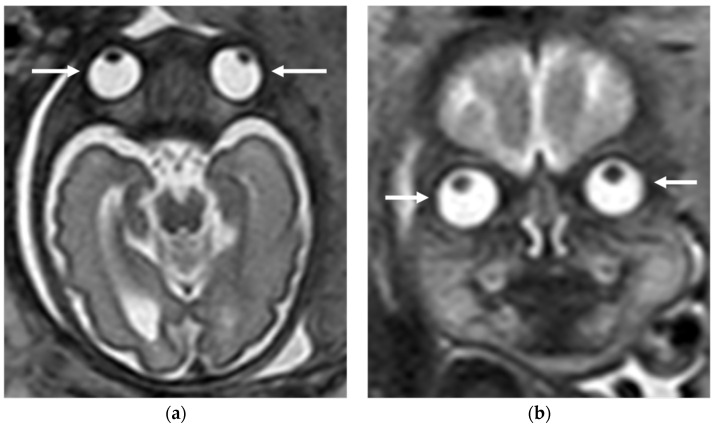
Fetal MRI of normal globes at 29th week of gestation: (a) Axial T2-weighted image and (b) coronal T2-weighted image showing normally formed bilateral symmetrical globes (white arrows).
